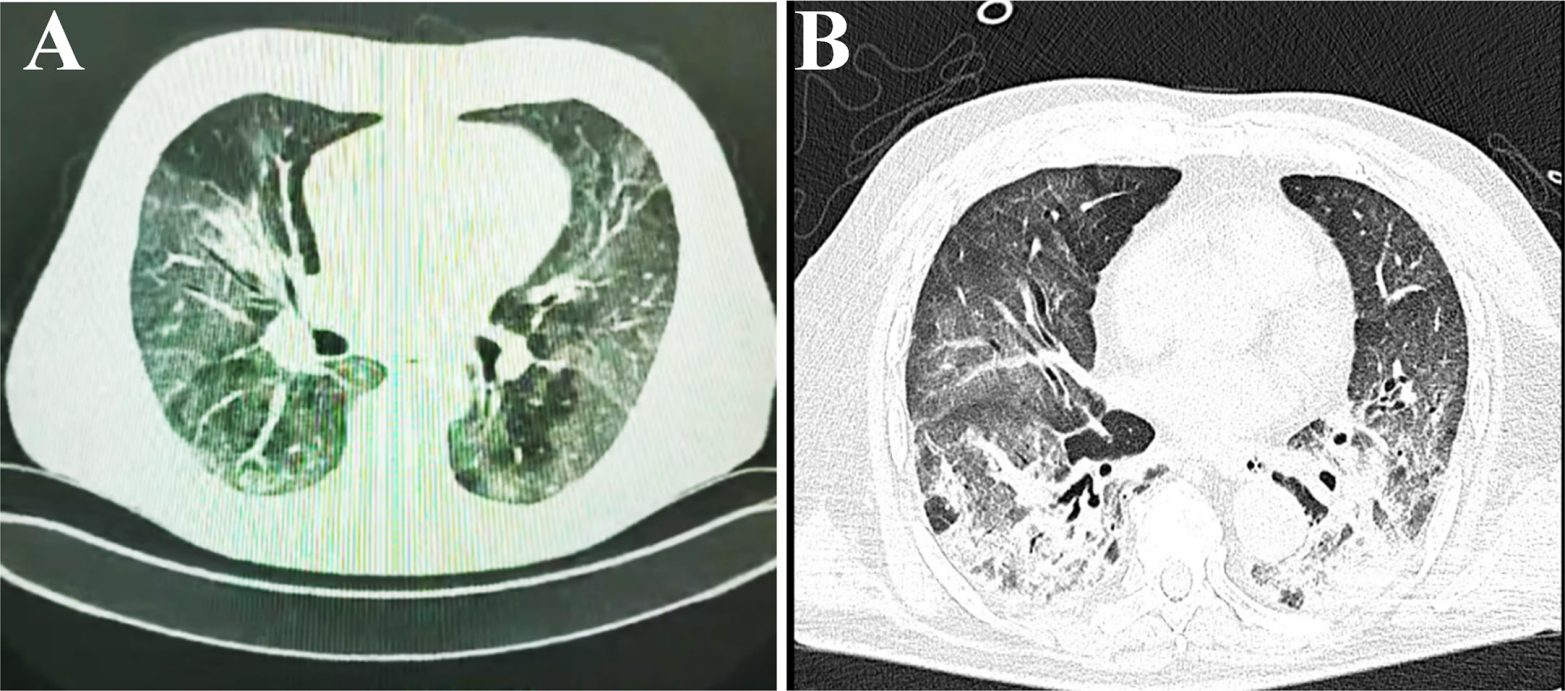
Chest computed tomography scan of two patients with critical illness due to COVID‐19. (A) A 58‐year‐old male, RT‐PCR positive for SARS‐CoV‐2, has a chest tomography scan with multiple ground‐glass lesions, reticulations, and condensation areas, CO‐RADS 6 classifications. (B) A 47‐year‐old male, RT‐PCR positive for SARS‐CoV‐2, has a chest tomography scan with ground‐glass opacities, areas of atelectasis, and bilateral parenchymal lesions, CO‐RADS 6 classifications.
